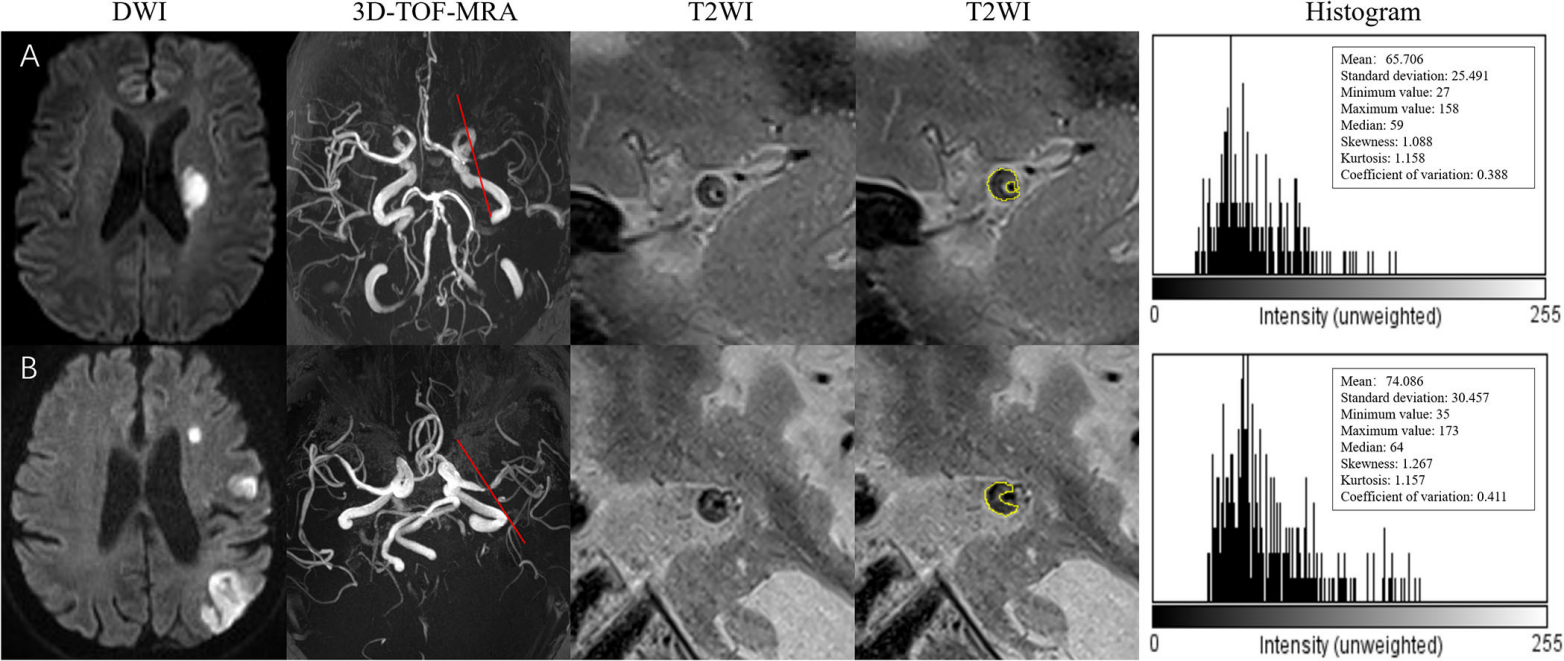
(A) A 73-year-old man presented with right-side weakness for 3 days. DWI showed an oval acute infarct in the left corona radiate. 3D-TOF-MRA demonstrated the stenosis located on the left MCA. The T2-weighted HRMRI demonstrated an eccentric atherosclerotic plaque with heterogeneity intensity at the most stenotic site of the right MCA. The outer wall boundary of the plaque can be outlined. The quantitative histogram parameters based on T2WI are shown on the right. (B) A 59-year-old man presented with right-side weakness and speech disorder for 5 days. DWI showed multiple infarctions in the left MCA territory. 3D-TOF-MRA demonstrated the stenosis located on the left MCA. The T2-weighted HRMRI demonstrated an eccentric atherosclerotic plaque with heterogeneity intensity at the most stenotic site of the right MCA. The outer wall boundary of the plaque can be outlined. The quantitative histogram parameters based on T2WI are shown on the right.
